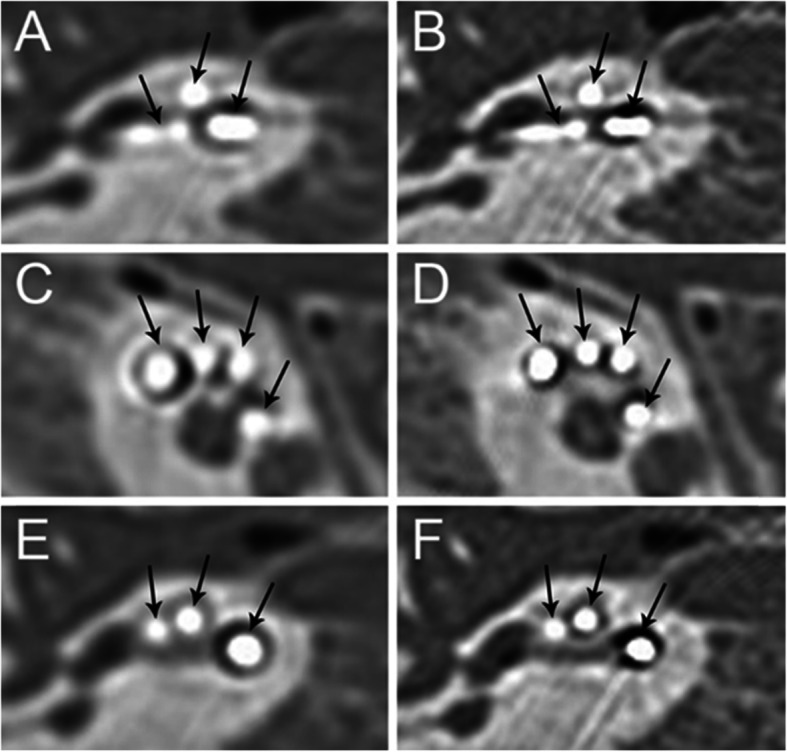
CT images of cochlear implants before and after using SEMAR. The image is greatly affected by electrode artifacts before using SEMAR, as part of the cochlea is obscured by the artifacts, and the electrode condition is displayed unsatisfactorily (a, c, e). The electrode artifacts in the image are reduced after using SEMAR, which allows clear observation of the corresponding structure and specific conditions of electrodes (b, d, f). The arrows in the figure indicate electrodes
